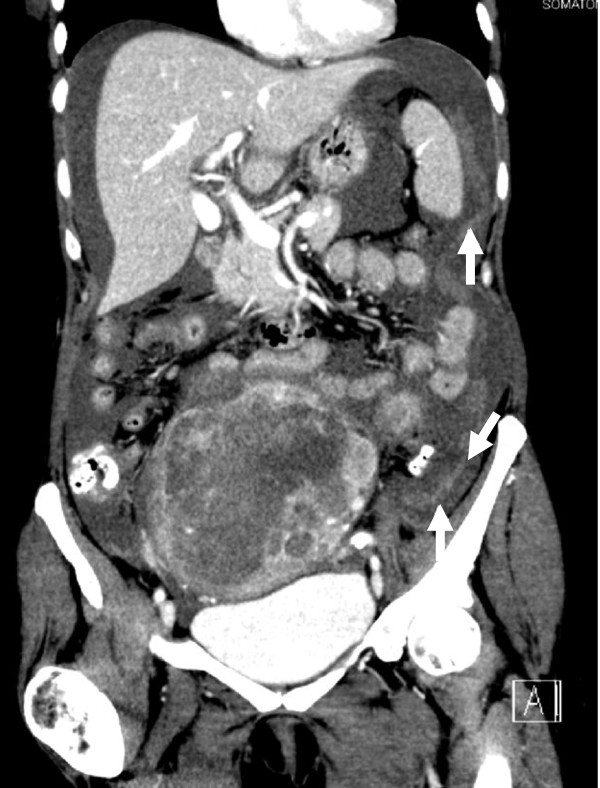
Abdominal computed tomography scan (A) 12 hours after first computed tomography scan showing diffuse intraabdominal bleeding (white arrows). There is increase in the free abdominal fluid and inhomogeneity of the abdominal mass.
